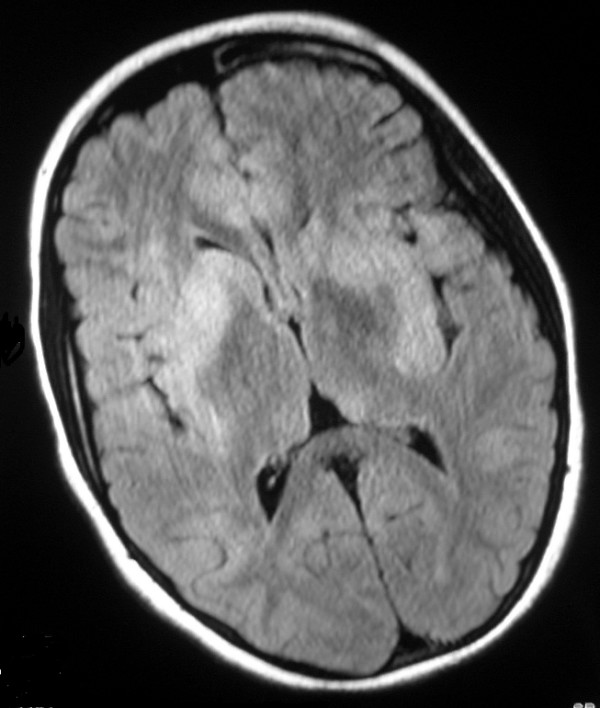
Axial FLAIR sequence magnetic resonance image of brain at admission. There are hyperintense multifocal lesions in the deep grey nuclei, subcortical white matter and cortex.
